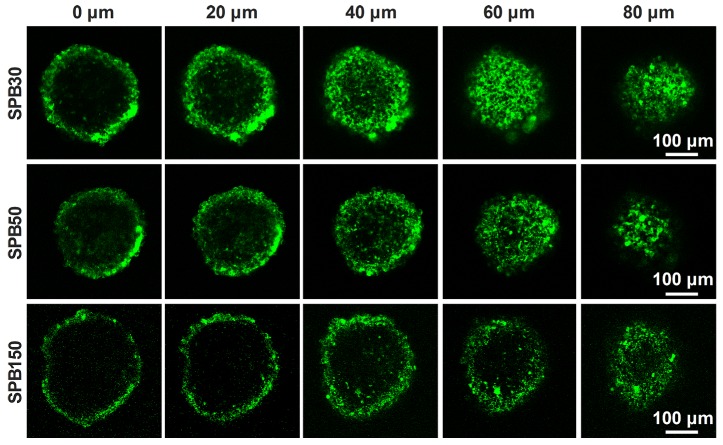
Representative Z-stack CLSM images of CT-26 MCTs incubated with FITC-labeled SPB NPs for 24 h were obtained starting in the middle of the spheroid in 20 μm intervals.
